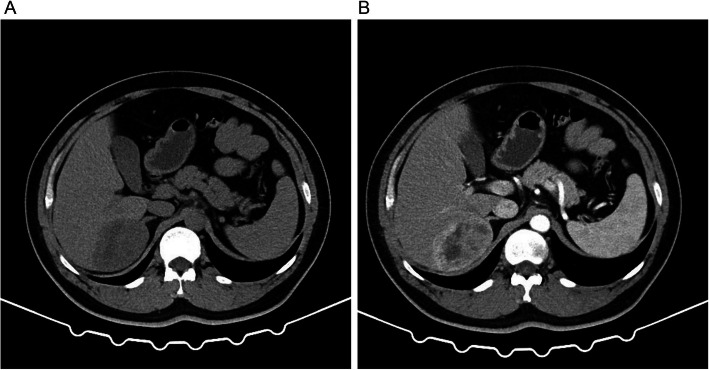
CT scanning of the mass. a A large mass was located between segment 6 of the liver and right adrenal gland. b In enhanced CT scanning, the margin of the mass displayed apparent enhancement in arterial phase
